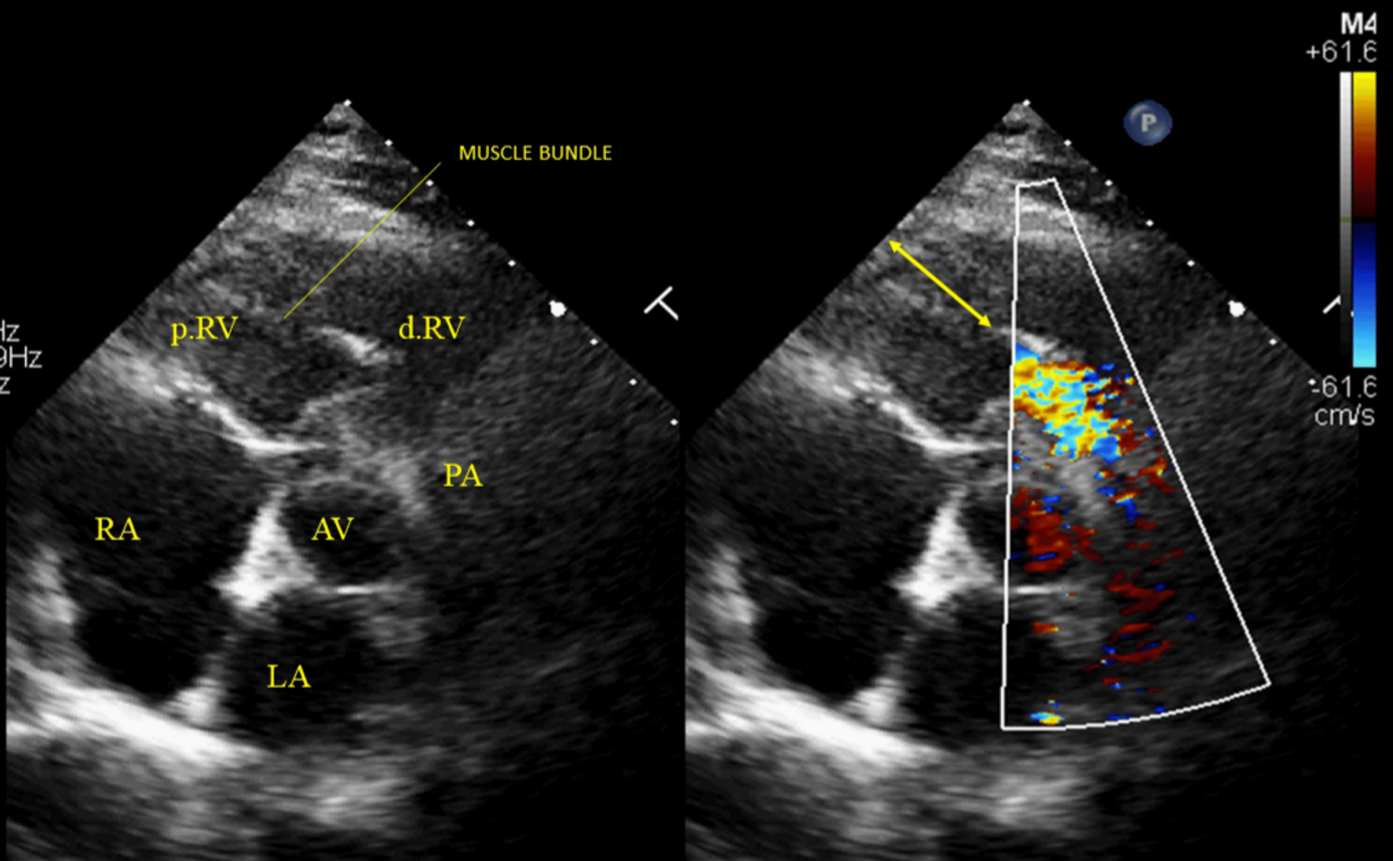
Two-dimensional transthoracic echocardiographic in the parasternal short axis at the aortic valve level Two-dimensional transthoracic echocardiogram images in the parasternal short-axis view at the level of the aortic valve during end-systole. The left panel shows the right ventricular cavity divided by hypertrophied muscular bundles. The right panel, with colour Doppler, demonstrates turbulent flow across the mid-right ventricle, consistent with a DCRV. AV: aortic valve, d.RV: distal low-pressure RV, LA: left atrium, PA: pulmonary artery, p.RV: proximal high-pressure RV, RA: right atrium, DCRV: double-chambered right ventricle
